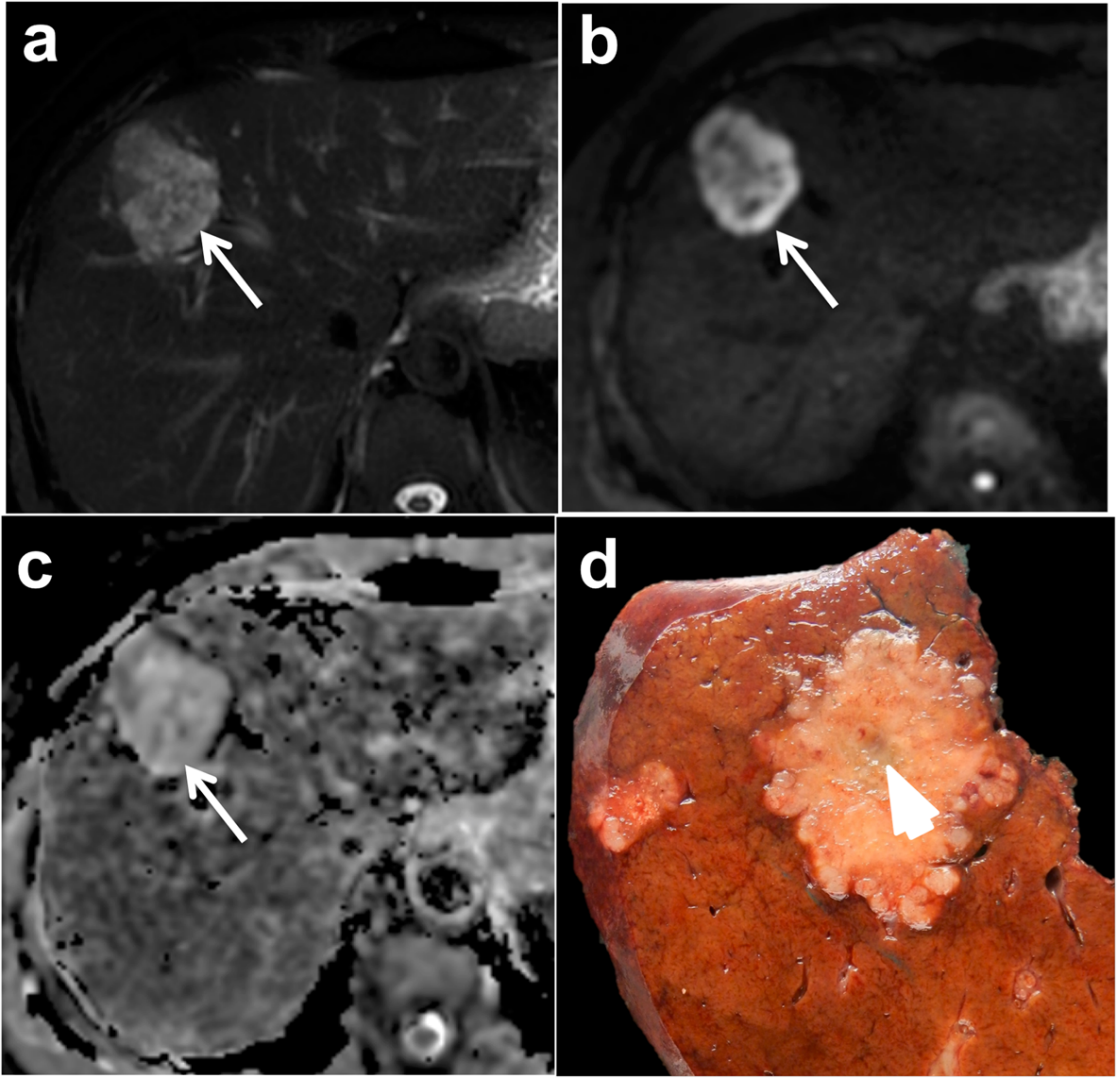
Example of mucinous regression of a metastasis of non-otherwise specified (NOS) adenocarcinoma of the rectum in a 52-year-old male patient treated by chemotherapy followed by hepatic resection. On preoperative MR imaging the lesion (arrows) shows high signal intensity on the fat-saturated fast spin echo T2-weighted image (a) and the diffusion weighted image (b 800 s/mm^2^) (b). The apparent diffusion coefficient was high (c). Gross pathology (d) shows a polylobated metastasis with central mucinous regression, containing lakes of acellular mucine (arrowhead)
